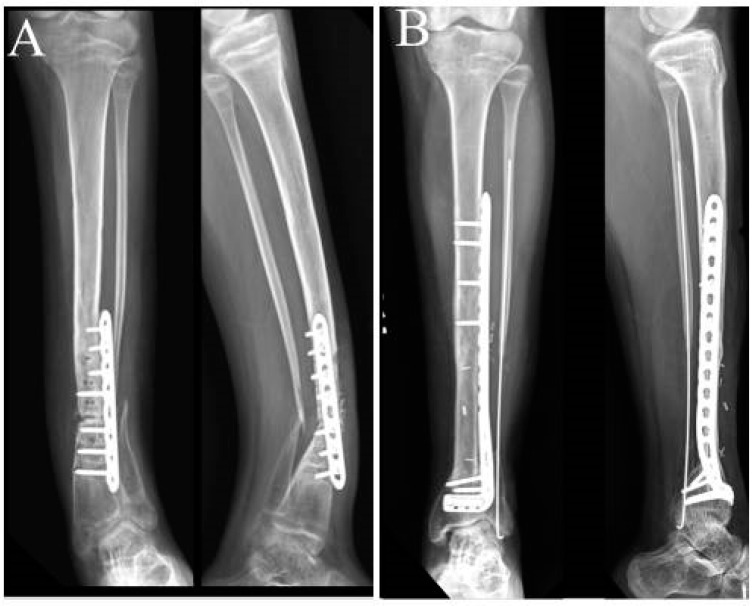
Radiographs of patient 3: (A) preoperative AP and lateral radiographs and (B) postoperative AP and lateral radiographs at 18 months after the surgery.
